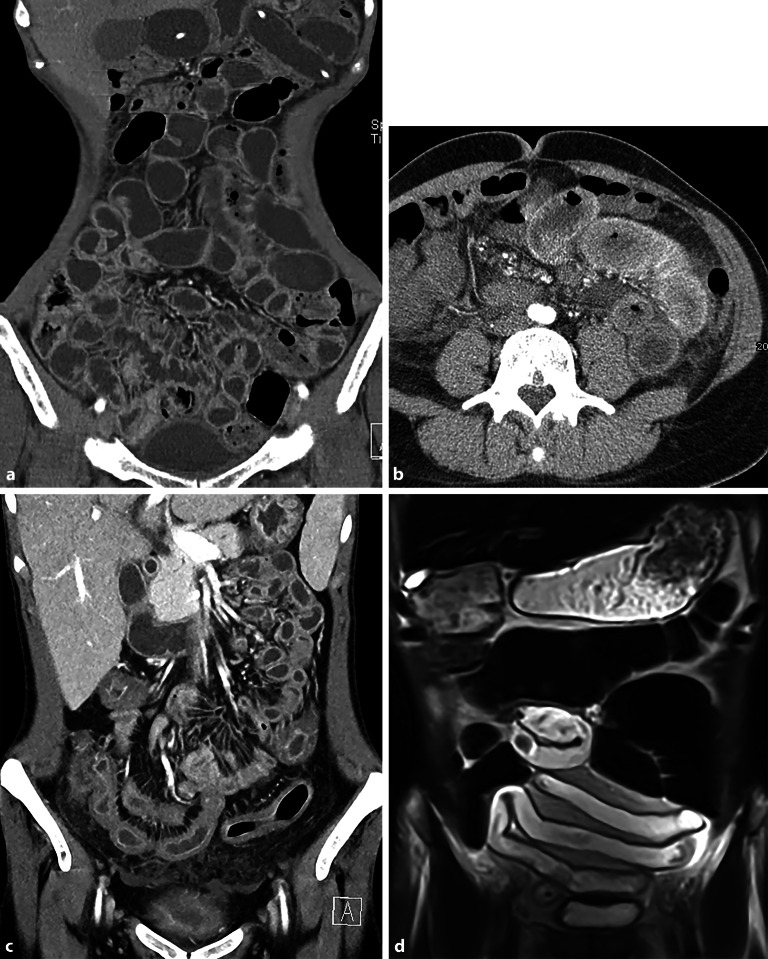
a CT(Computertomographie)-Enteroklysma zeigt eine Zöliakie mit Faltenverlust jejunal und Faltenvermehrung ileal; b CT arteriell axial: serosale Form einer eosinophilen Enteritis mit verstärkt serosalem Kontrastmittel-Enhancement und mesenterialem Ödem; c CT venös koronal: akute GvHD („graft-versus-host disease“) mit flüssigkeitsmarkierten Dünndarmschlingen und deutlich hypervaskularisierter Darmwandverdickung; d MR(Magnetresonanz)-Enterographie mit dem chronischen Bild einer GvHD mit Faltenverlust und bandförmig angeordneten Dünndarmschlingen
